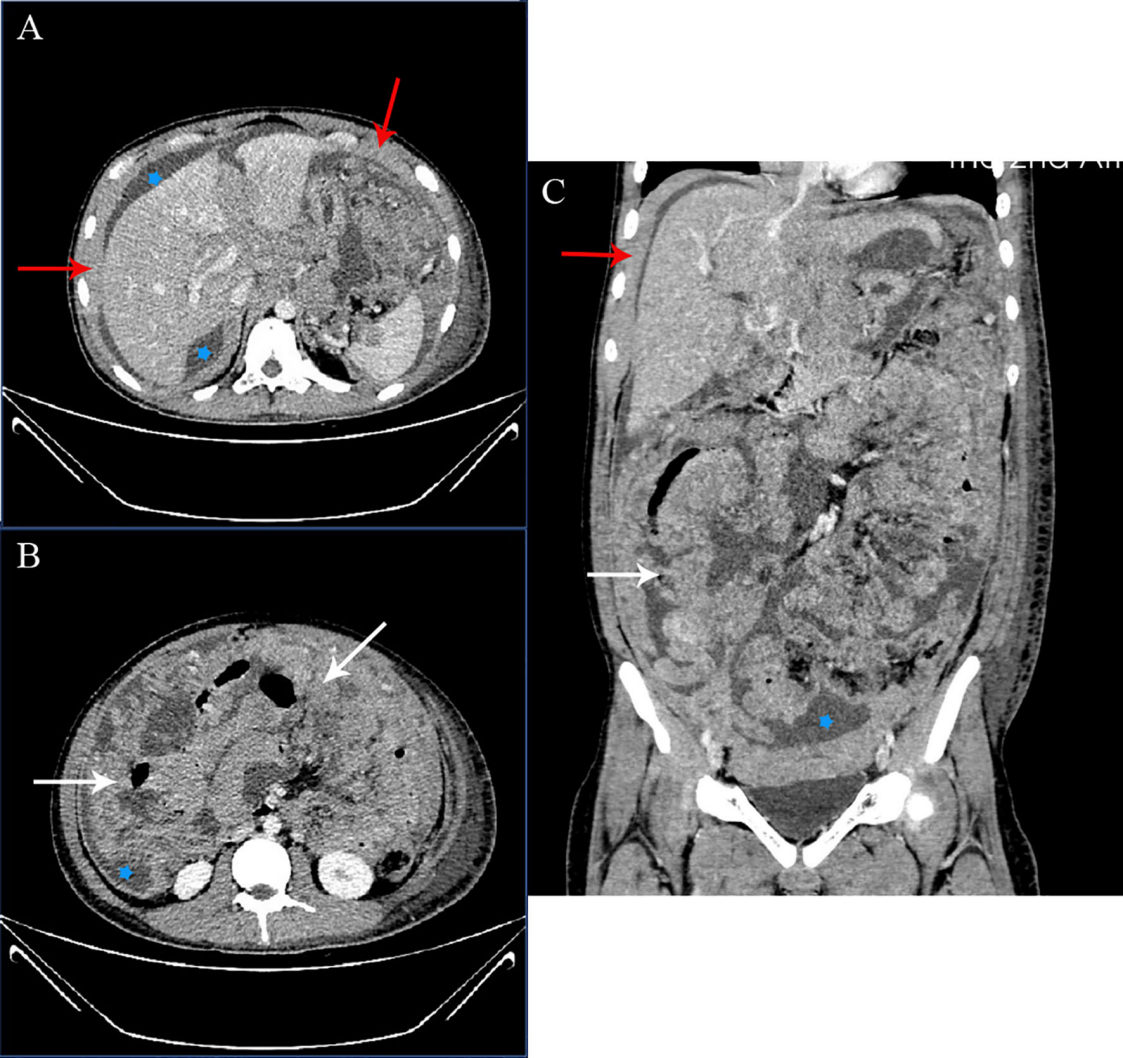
CT examination before-treatment. Axial (A), Axial (B), and coronal (C) enhanced CT images of the abdomen and pelvis in the portal phase. Extensive ascites (stars), thickening of the peritoneum (red arrow), and marked thickening of the omentum (white arrows) noted. No enlarged lymph nodes were noted.
